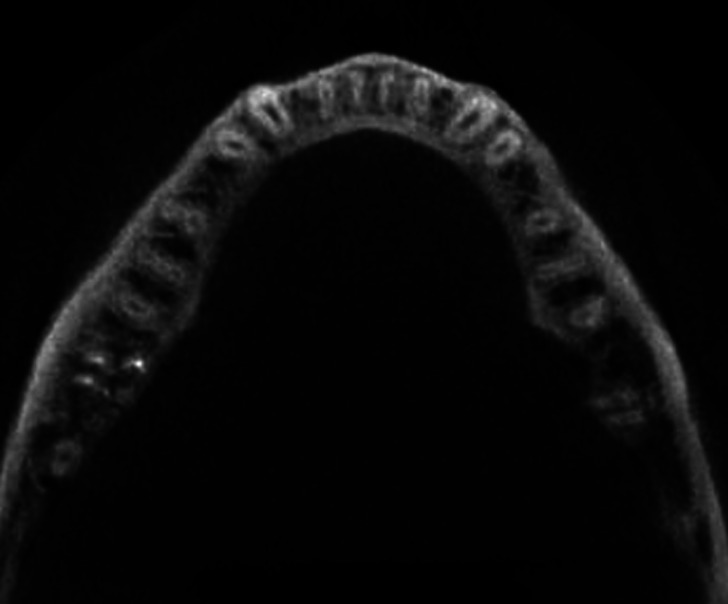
CBCT axial image of 4-rooted second mandibular molars present bilaterally.
